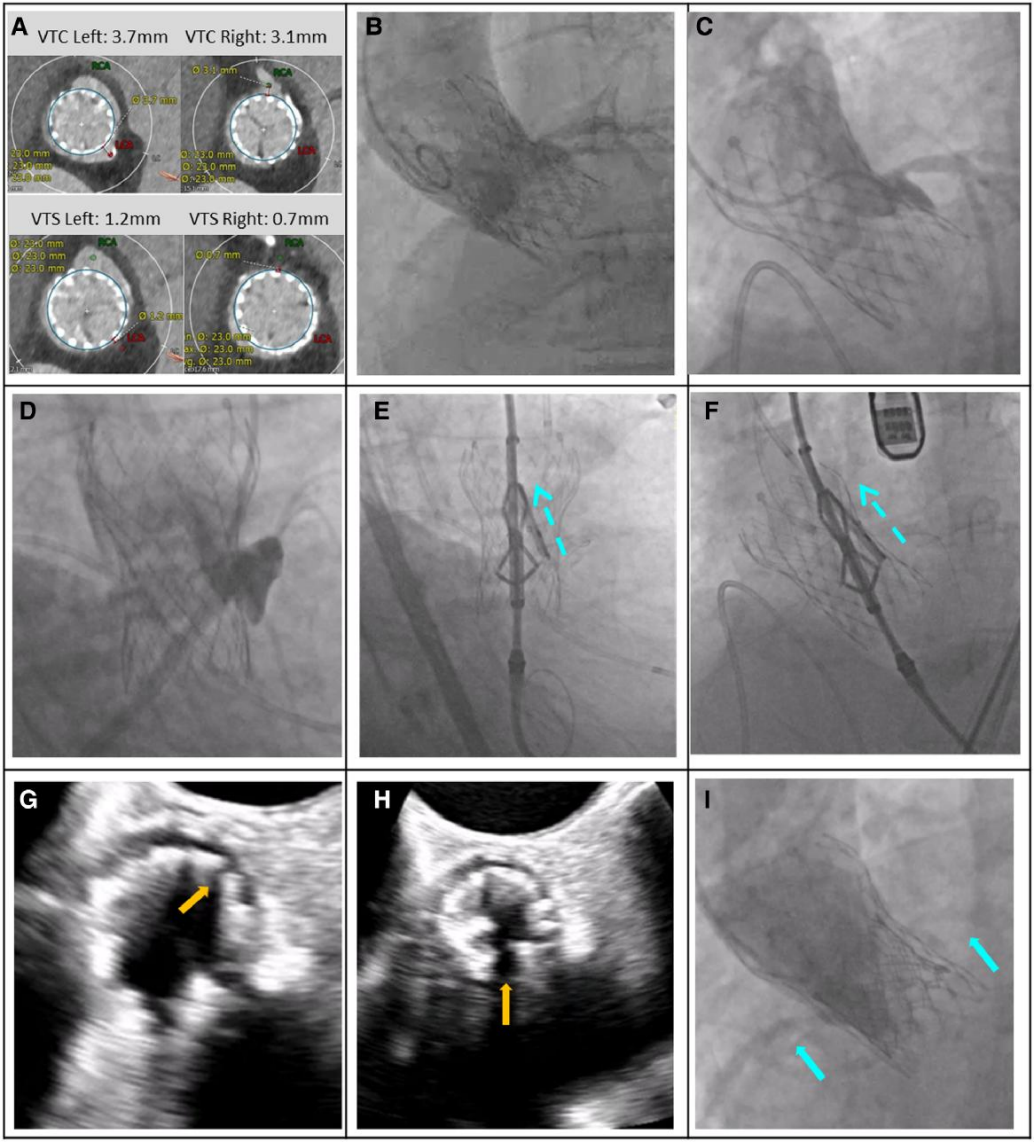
A ShortCut procedure in a patient with a failed transcatheter heart valve. A 79-year-old female with a failed Evolut R 29 mm transcatheter aortic valve (Medtronic) at high risk for double coronary obstruction due to leaflets extending well above the sinotubular junction with effaced sinuses and narrow sinotubular junction, that was treated with ShortCut and was implanted with a 23 mm SAPIEN 3 valve (Edwards Lifesciences). (*A*) Computed tomography measurements showing predicted risk of coronary obstruction. (*B–D*) Baseline aortogram, right and left coronary angiographic demonstrating of the risk for coronary obstruction (side view of left and right coronary cusps). (*E* and *F*) The ShortCut is positioned. Activated splitting element punctures the leaflet from the ventricular side just above the annulus. The leaflet is split by gently retracting the catheter while feeding the guidewire. (*G* and *H*) Echocardiography verifies left and right leaflet split (annotated arrows). (*I*) Post-TAVI demonstration of normal coronary flow (annotated arrows). Echocardiography post-TAVI (not shown) showed aortic valve gradients of 11/5 mmHg. The patient had an uneventful hospital stay
